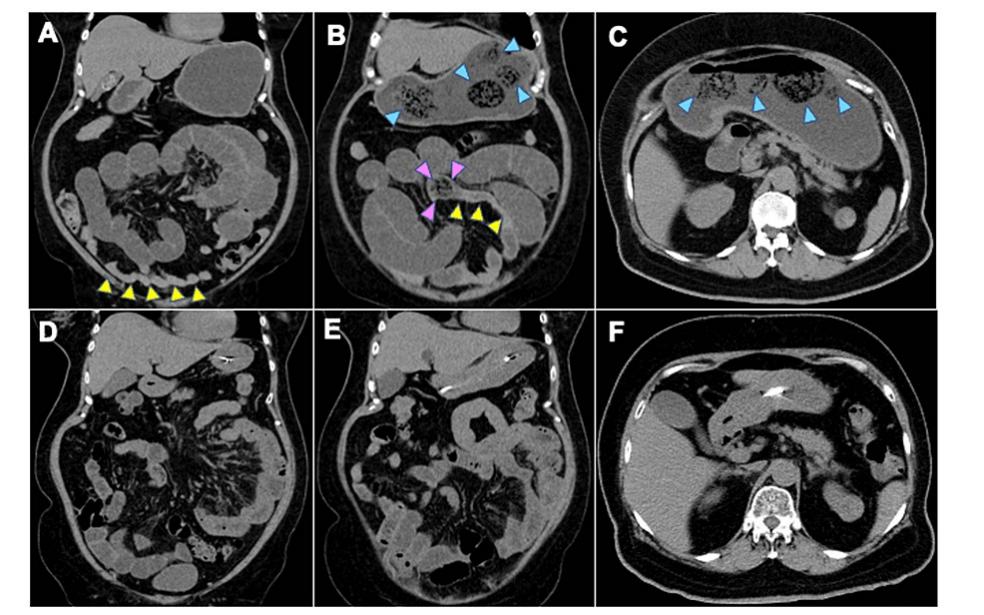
Abdominal computed tomography showing small bowel obstruction due to the impaction of an enterolith. Dilatation of the proximal small bowel loops and collapse of the distal small bowel loops (yellow arrowheads) are observed (A, B). An enterolith (3 cm in diameter; pink arrowheads) is present at the abrupt transition point between the dilated and collapsed small bowels (B). Multiple bezoars (blue arrowheads) are detected in the stomach (B, C). Resolution of the small bowel obstruction was achieved after the treatment (D, E, F), accompanied by the expulsion of the enterolith and gastric bezoars. Neither enteroliths causing small bowel obstruction nor gastric bezoars were detected.
